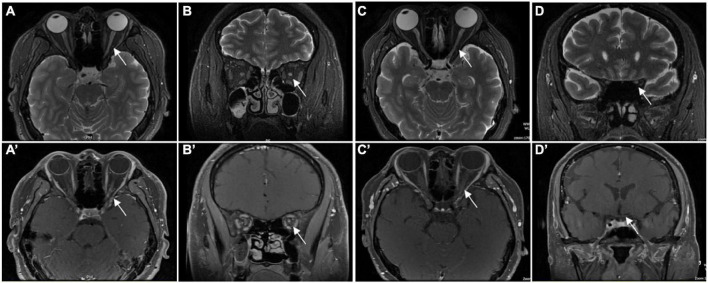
Orbital MRI images in patients who were diagnosed with ON. Panels (A,C) (axial) and (B,D) (coronal) showed the high signal of the left ON (the white arrow) in T2WI. Panels (C) (axial) and (D) (coronal) showed the enhancement of left ON (the white arrow) in gadolinium-enhanced T1. Panels (A′) (axial) and (B′)(coronal) showed the enhancement of left ON (white arrow) in gadolinium enhanced T1. Panels (C′) (axial) and (D′) (coronal) showed the normal signal of left ON (the white arrow) in gadolinium-enhanced T1.
